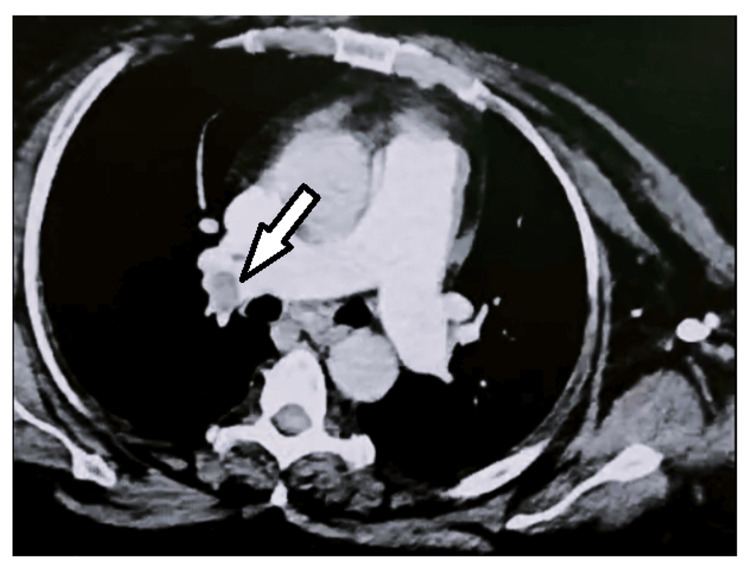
CT pulmonary angiography showing bilateral PE. Axial CTPA image demonstrating filling defects in the right main pulmonary artery and left lower lobe pulmonary artery, consistent with bilateral pulmonary embolism. The arrow indicates an obvious thrombus within the right pulmonary artery. PE: pulmonary embolism; CTPA: computed tomography pulmonary angiography
